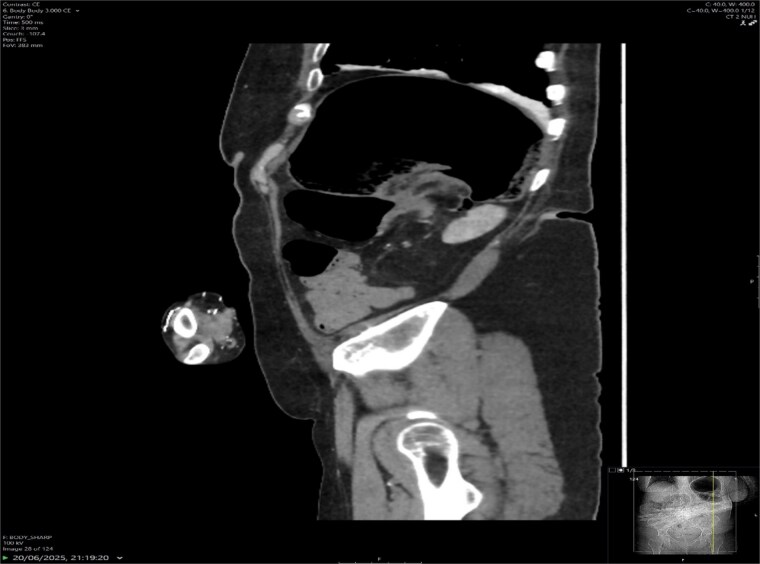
Sagittal CT showing swirl sign and dilated large bowel loops.
